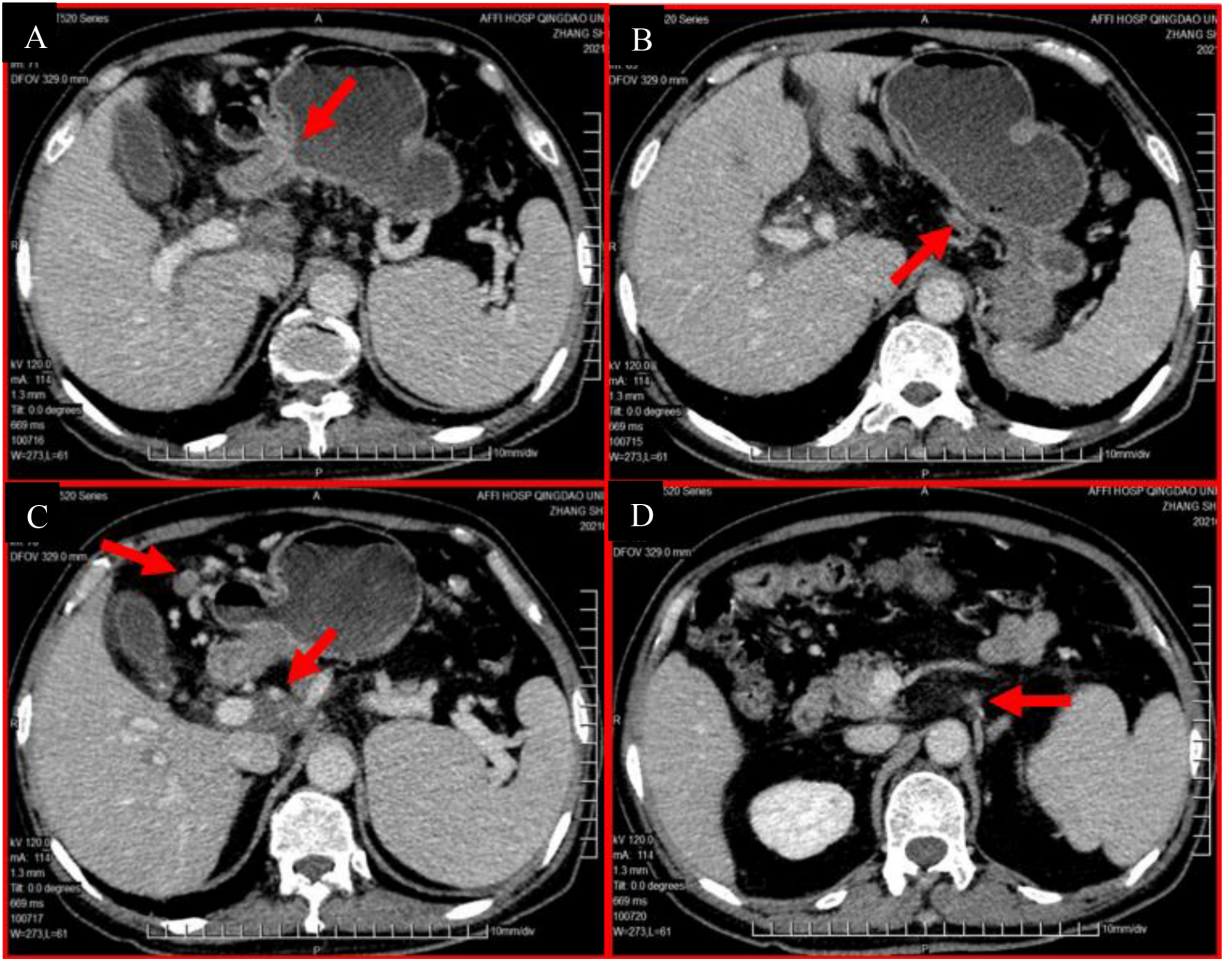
Restaging contrast-enhanced abdominal CT after completion of 6 cycles of conversion therapy (C6). (A-D).
